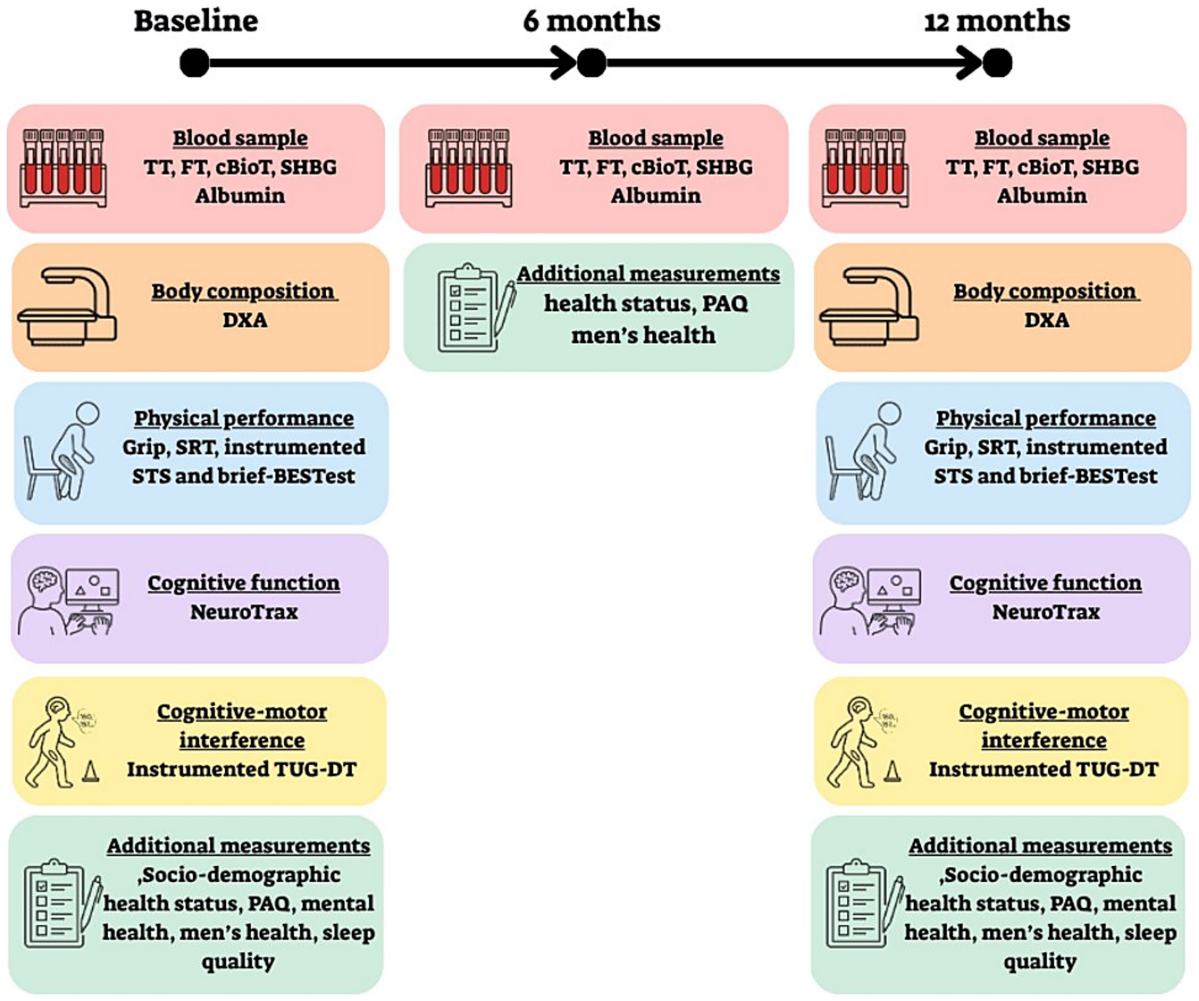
Description of the assessments to be performed at the three study time points (baseline, 6 months, and 12 months). TT, Total testosterone; FT, free testosterone; cBioT, Calculated bioavailable testosterone; SHBG, Sex hormone−binding globulin; DXA, Dual-energy X-ray Absorptiometry; SRT, Sitting-rising test; STS, Sit-to-stand; brief-BESTest, brief-balance evaluation systems test; NeuroTrax- computerized cognitive tests; TUG-DT, Timed up and go with dual task; PAQ, Physical activity Questionnaire.
